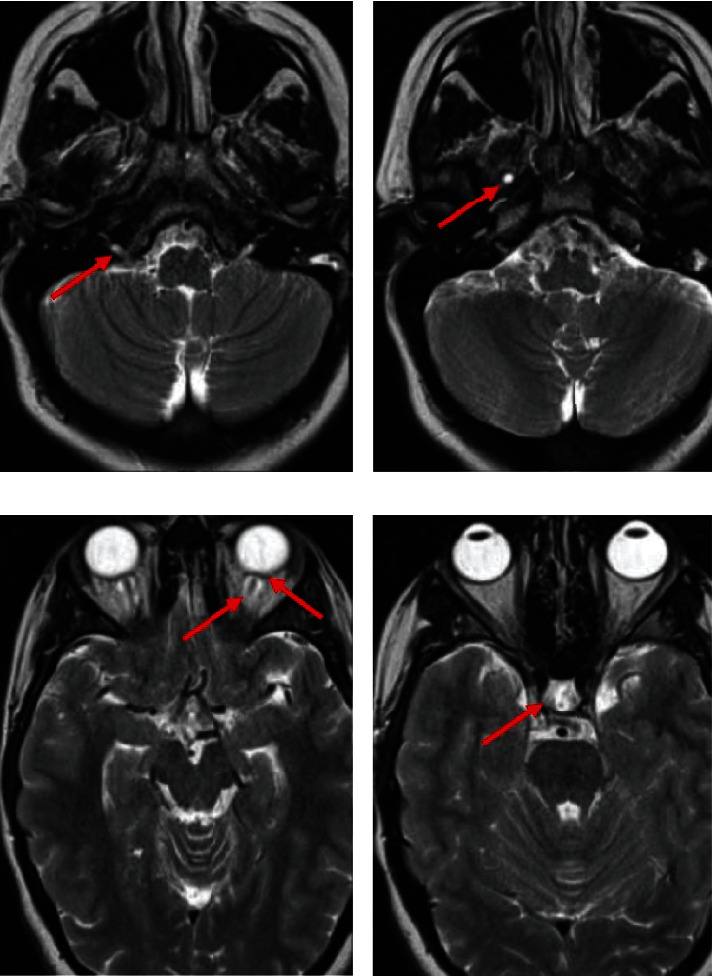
Magnetic resonance imaging findings: enlarged hypoglossal canal (a), small petrous apex meningocele (b), perioptic nerve sheath distension and flattening of the posterior ocular globe (c), and enlarged cerebrospinal fluid filled pituitary fossa (“empty sella”) (d).
